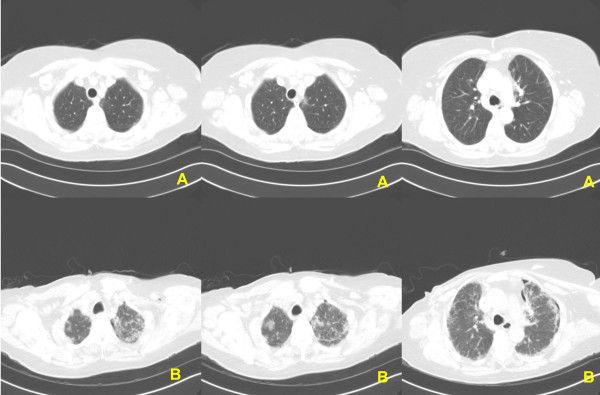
Computed tomography scans before (A), and (B) after the third cycle of docetaxel/cyclophosphamide chemotherapy
